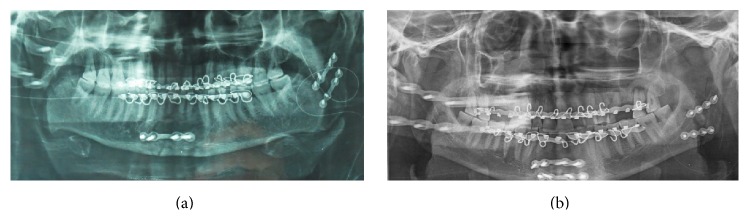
Postoperative check radiographs showing fixation of mandibular ramus fracture by two noncompression mini plates.
